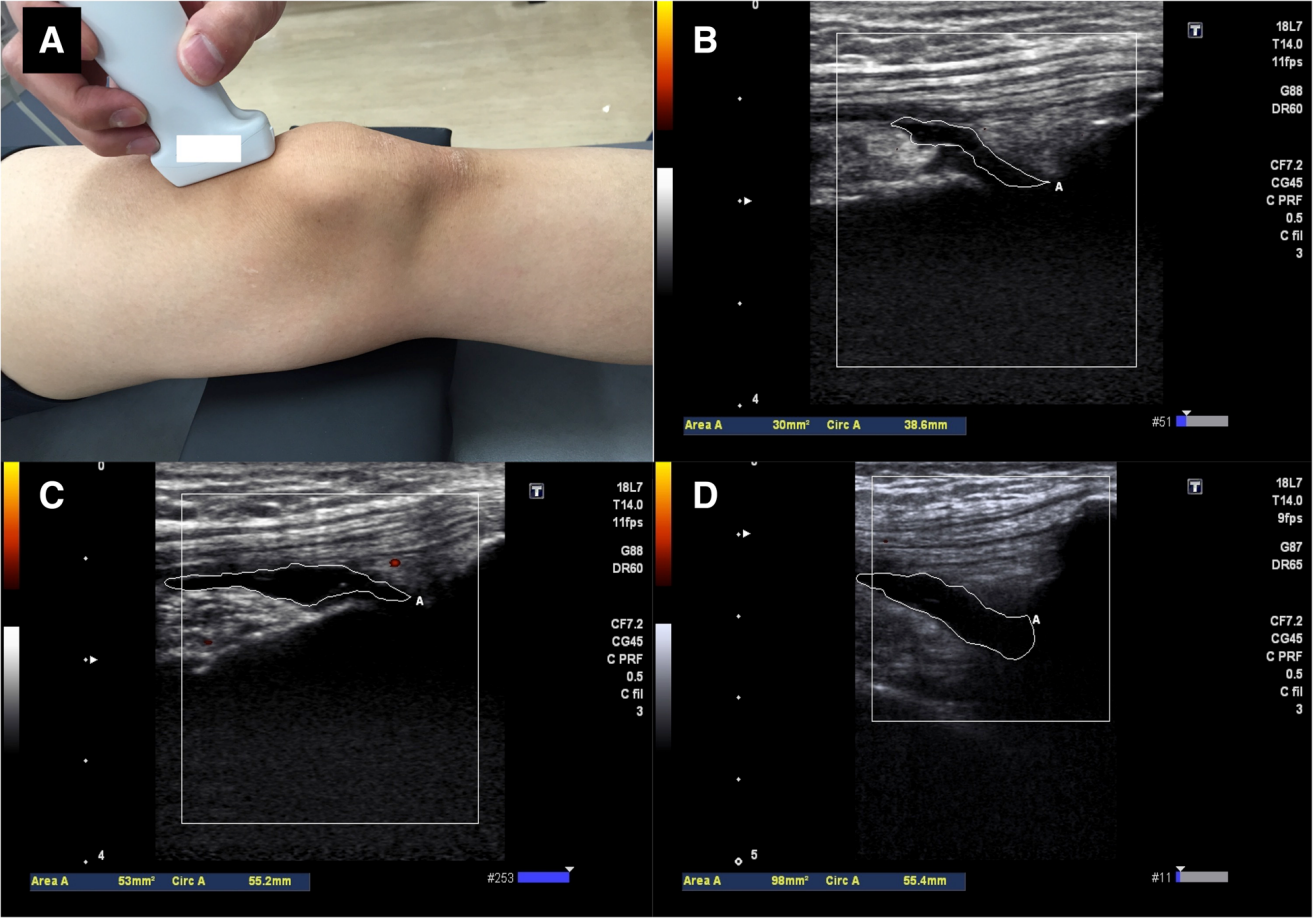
Representative images of the suprapatellar effusion area. a Ultrasound scan image of the suprapatellar effusion acquired by placing a linear probe longitudinally on the suprapatellar pouch. The effusion area was calculated by tracing the margin of the echo-free space that corresponds with the suprapatellar pouch. During the examination, the transducers should be placed on the subject as gently as possible to avoid distorting the acquired area. Representative images of the suprapatellar effusion area: 30 mm^2^ (b); 50 mm^2^ (c); and 100 mm^2^ (d)
